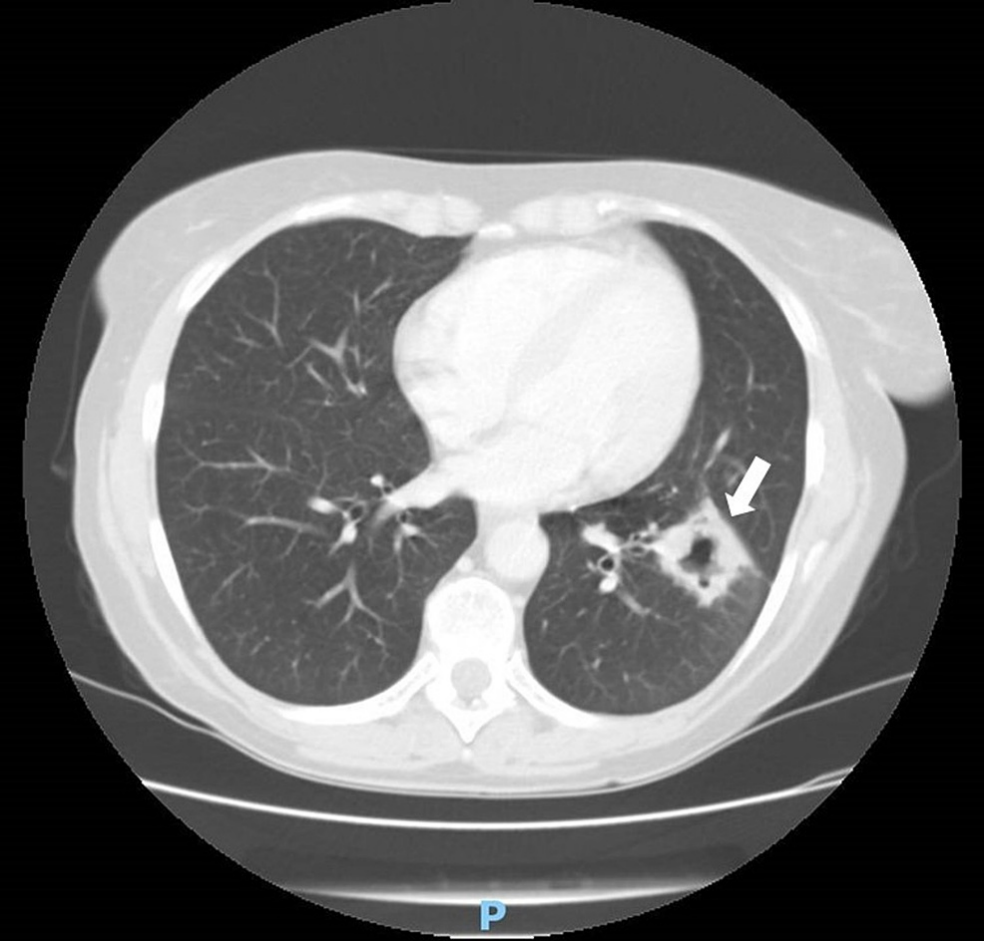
Thoracic CT scan showing rounded mass within a cavity, typical of aspergilloma (arrow)
